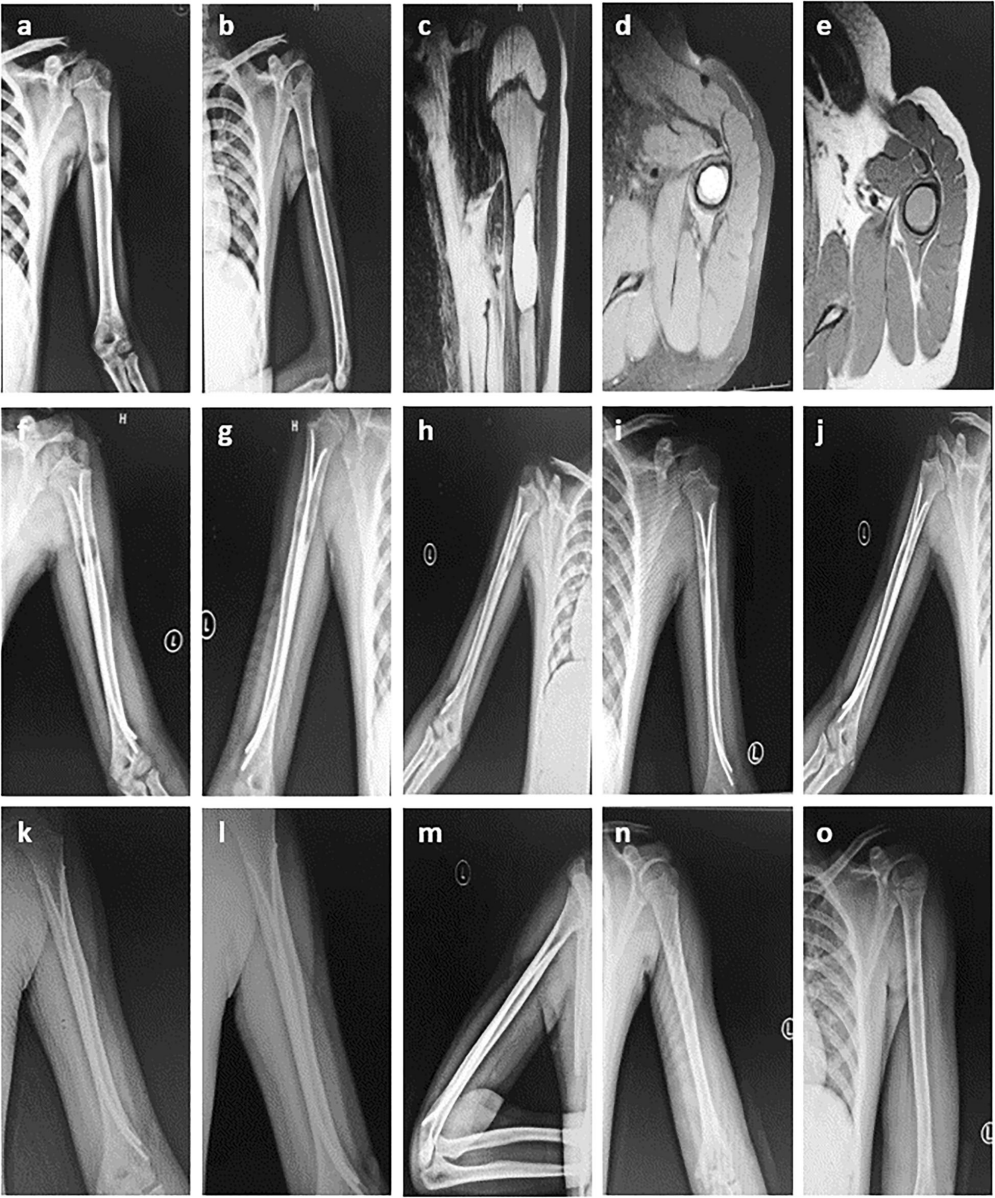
Radiographs of a 10-year-old boy who presented with pathological fracture of the left humerus. a-b Anteroposterior and lateral radiograph on admission. c-e MRI indicates a low signal on T1-weighted images and a homogeneous high signal on T2 weighting. f-k After the operation of minimally invasive intramedullary decompression and drainage with ESIN combined with intralesional injections of steroids; At the interval points of postoperative 1 weeks, 2 months, 6 months, 9 months, 12 months, 14 months, the lesion is significantly smaller and shows signs of healing gradually. At 14 months, Radiograph show complete cyst healing (Capanna grade 1 healing). l-o At 16 months, the bone cyst has resolved, and then the intramedullary nails are removed. n-o Radiographs show complete cyst healing (Capanna grade 1 healing) after second surgery
